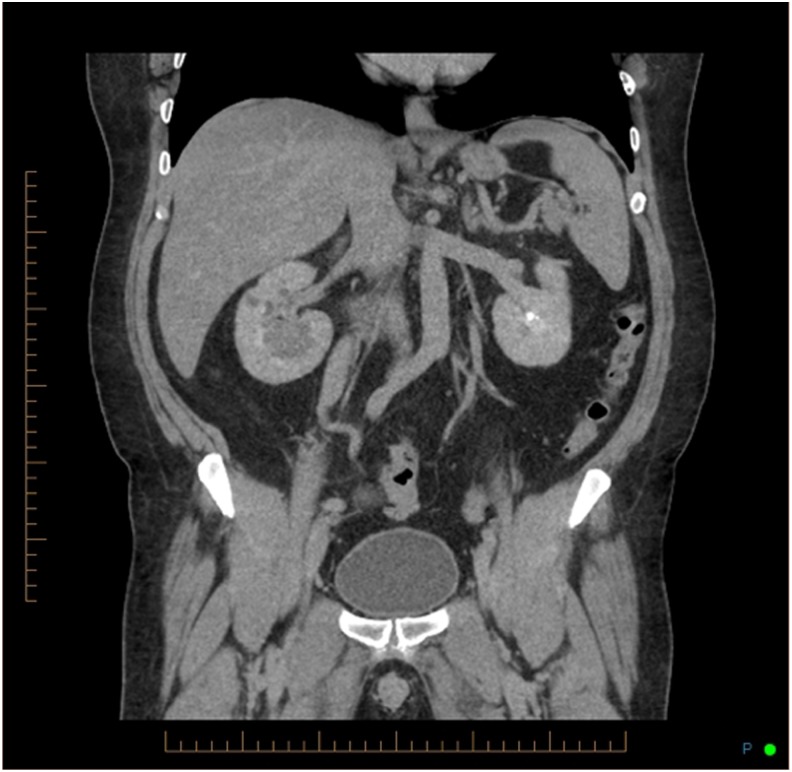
Coronal noncontrast CT scan image postendoscopic removal of the impacted right midureteral stone demonstrating residual chronic right hydroureteronephrosis and a left lower pole caliceal stone.
